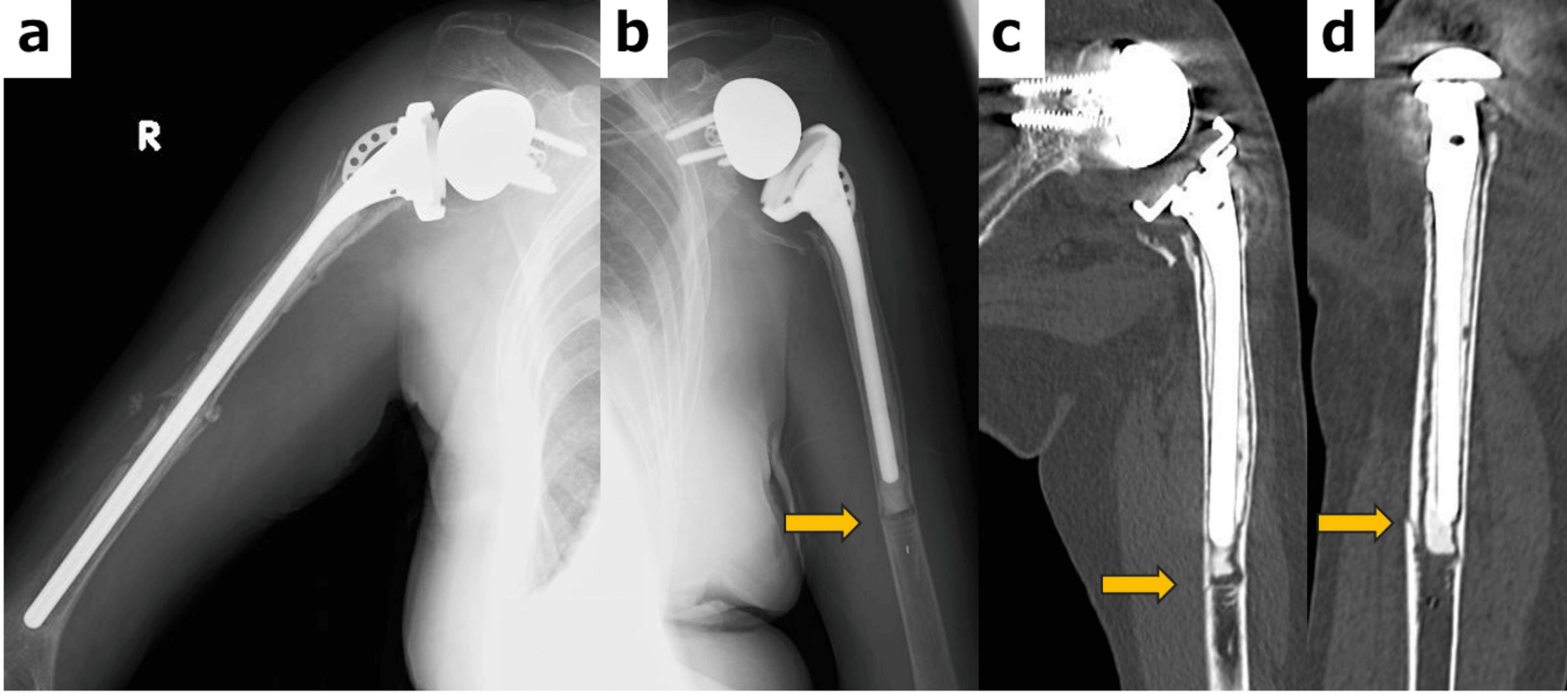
Radiographic findings at three months after right revision reverse shoulder arthroplasty and after the onset of pain in the left shoulder following reverse shoulder arthroplasty (RSA) (a) Three months after revision surgery. (b-d) A periprosthetic fracture at the distal tip of the humeral stem is visible on both X-ray and CT images at the location indicated by the arrows, accompanied by a radiolucent line around the cement mantle.
